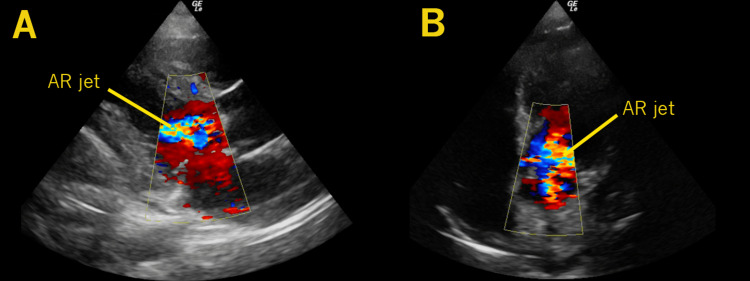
Aortic Regurgitation A parasternal long-axis view is seen with color Doppler over the aortic valve, demonstrating a turbulent aortic regurgitant jet (AR jet) (A). A similar finding is seen from the apical 5-chamber view (B).
